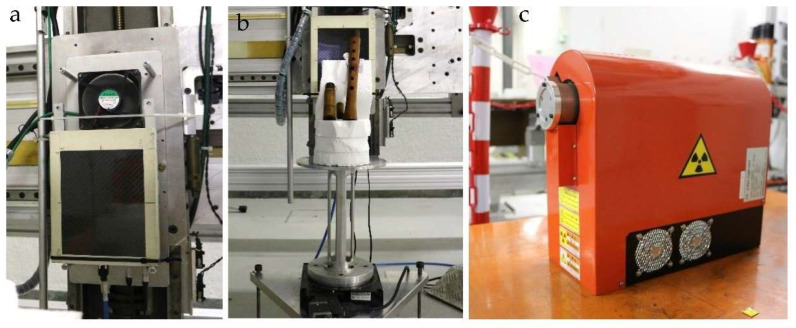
Experimental setup: (a) Flat-panel detector, (b) sample on the rotating platform, (c) X-ray source.
